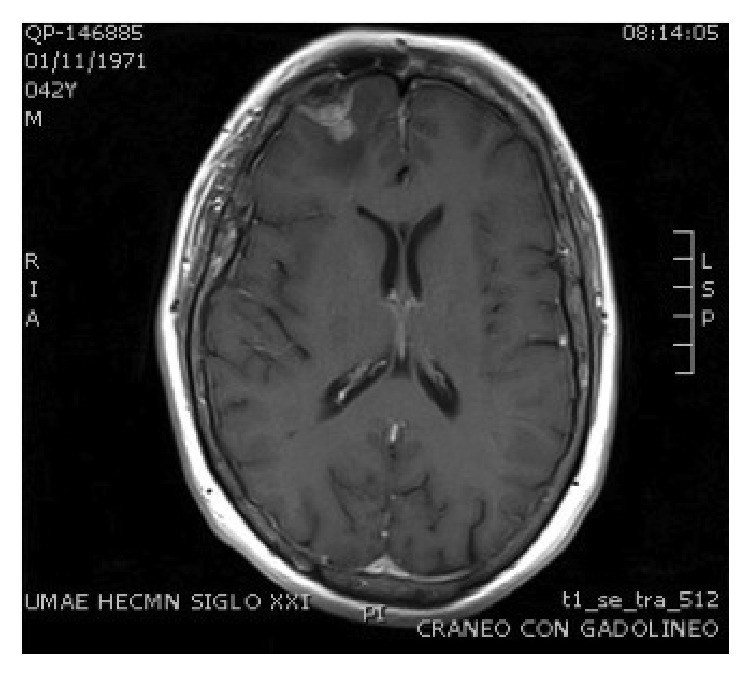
Brain MRI. Image in T1 shows a right frontal tumor of 19 × 21 × 19 mm with associated edema.
